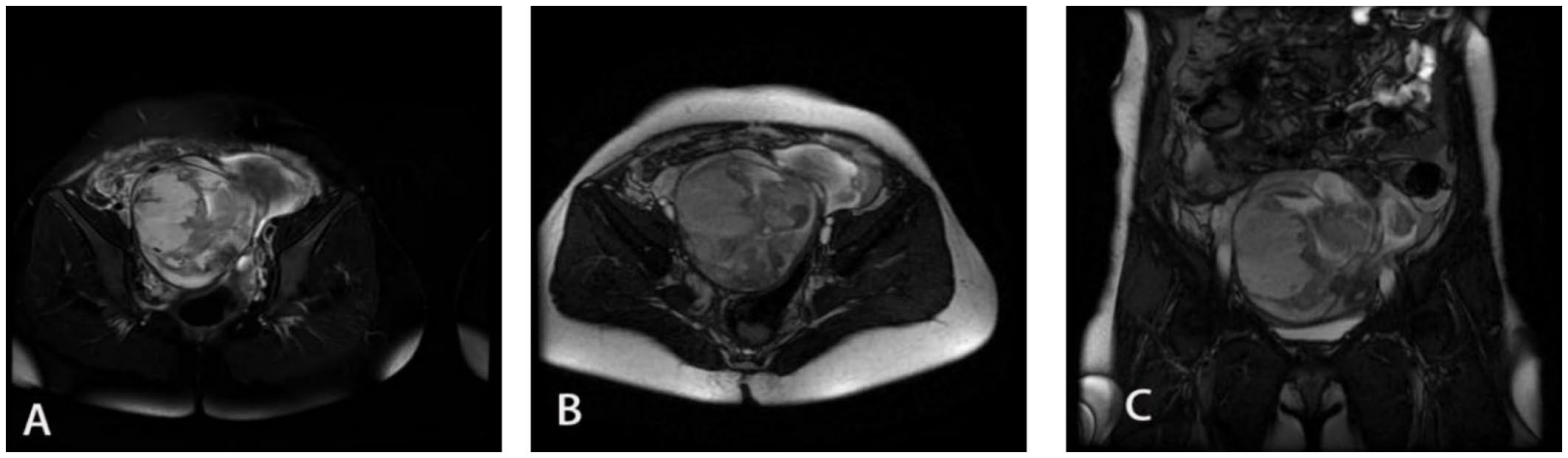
MRI images of Douglas pouch pregnancy of the fourth patient. The three pictures (A–C) show different sections of the lesion area of the fourth patient.
